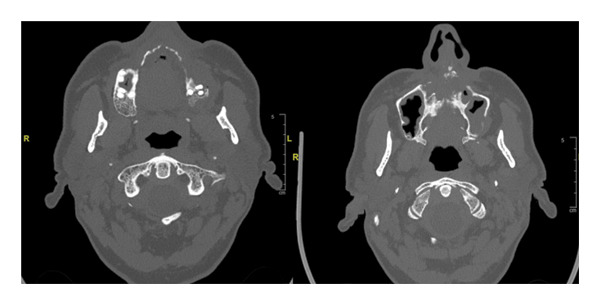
CT scan at 2 months postoperatively showing no disease progression.
